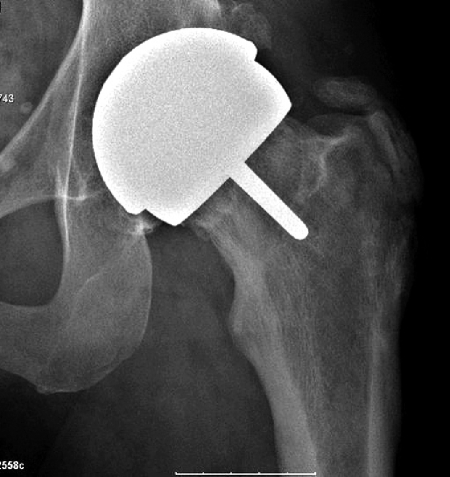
Osteonecrotic hip without necrotic signs on plain radiographs, 1 year after surgery.
